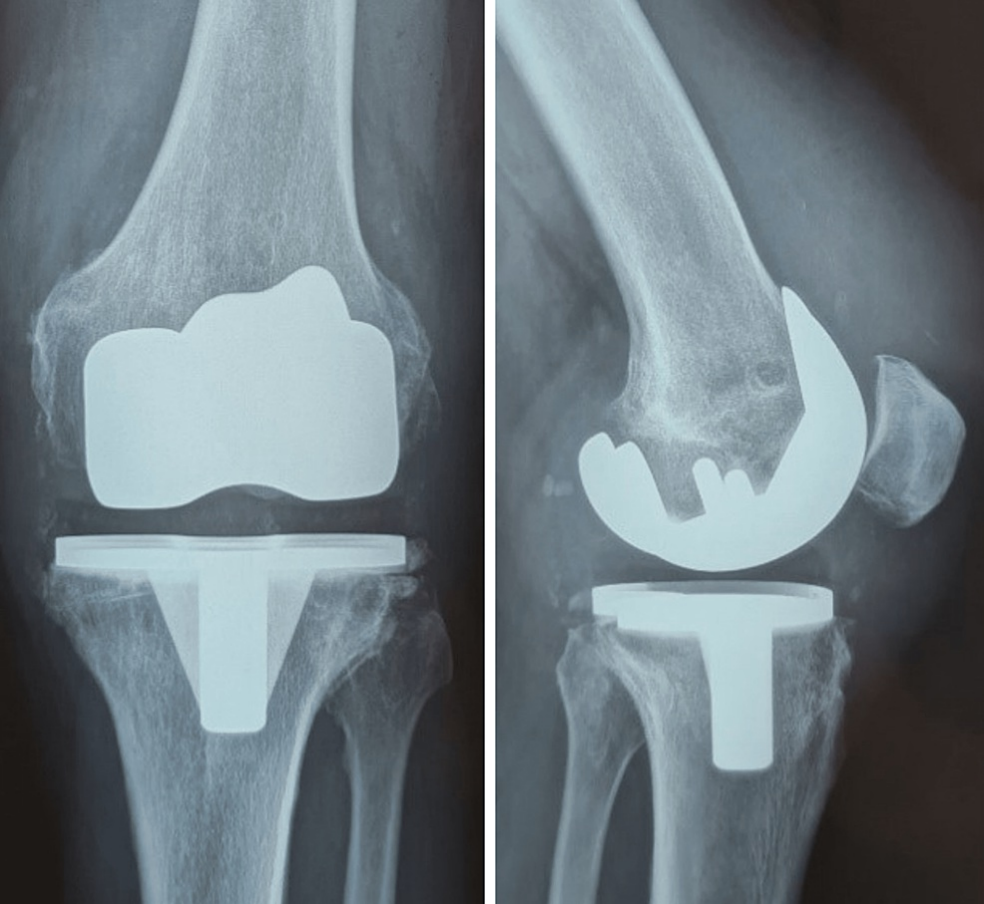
Antero-posterior (AP) and lateral (L) knee radiograph of the primary TKR
